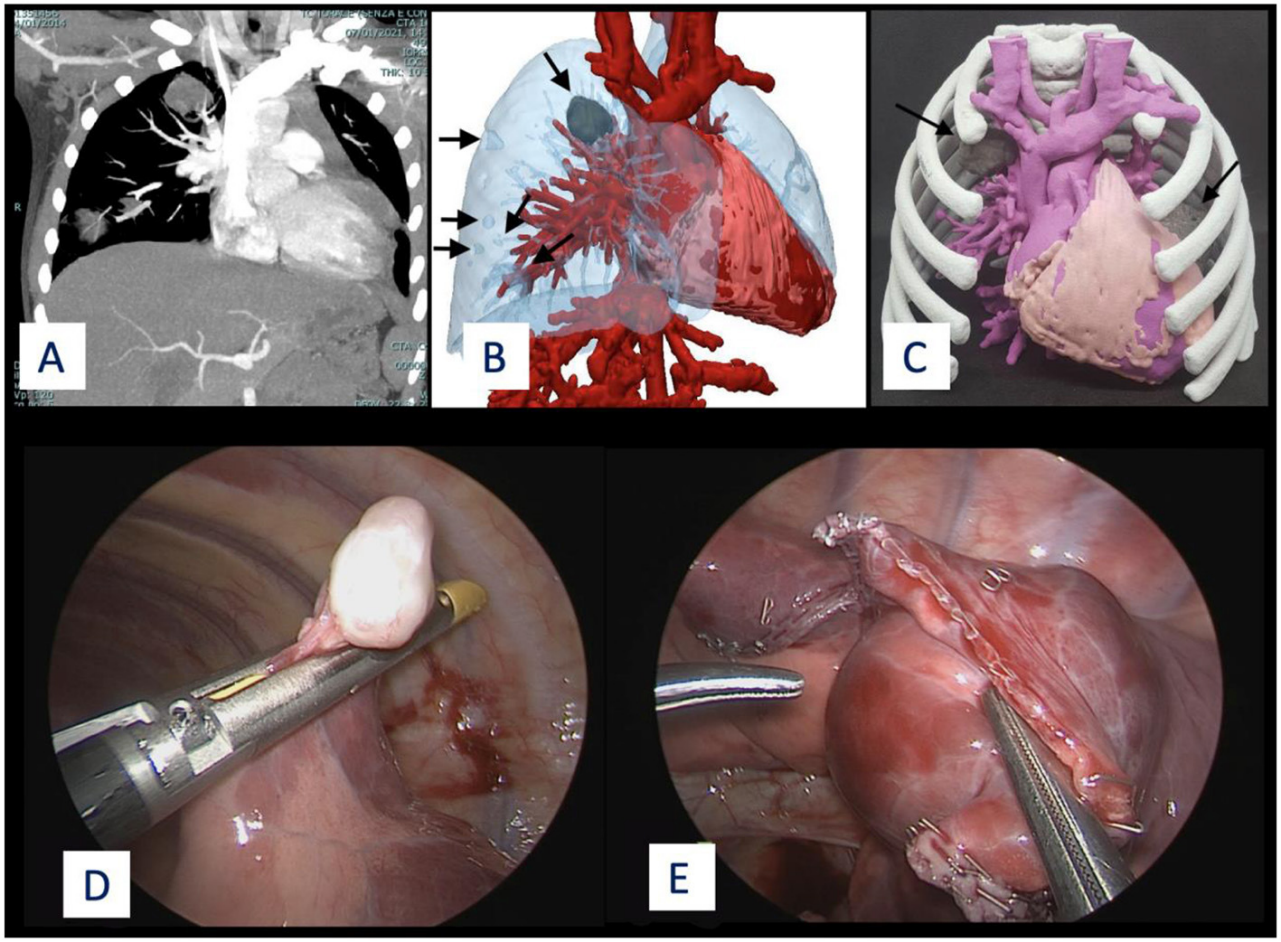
Multiple metastases of immature teratoma in a 7-year-old girl. Left single metastasis was excised through an open procedure, due to dimension and site of the mass. The remaining lesions (*n* = 6, one solid, five cystic ones) on the right lobe were excised through VATS. (A) CT image. (B) 3D virtual reconstruction. (C) 3D printed model showing the two solid lesions (colored in gray). (D,E) Intraoperative view.
